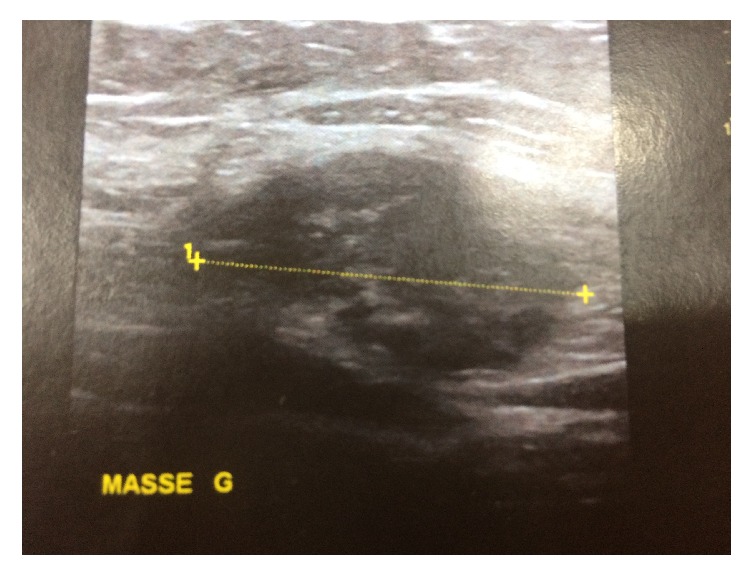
Heterogenous hypoechoic mass at sonography.
